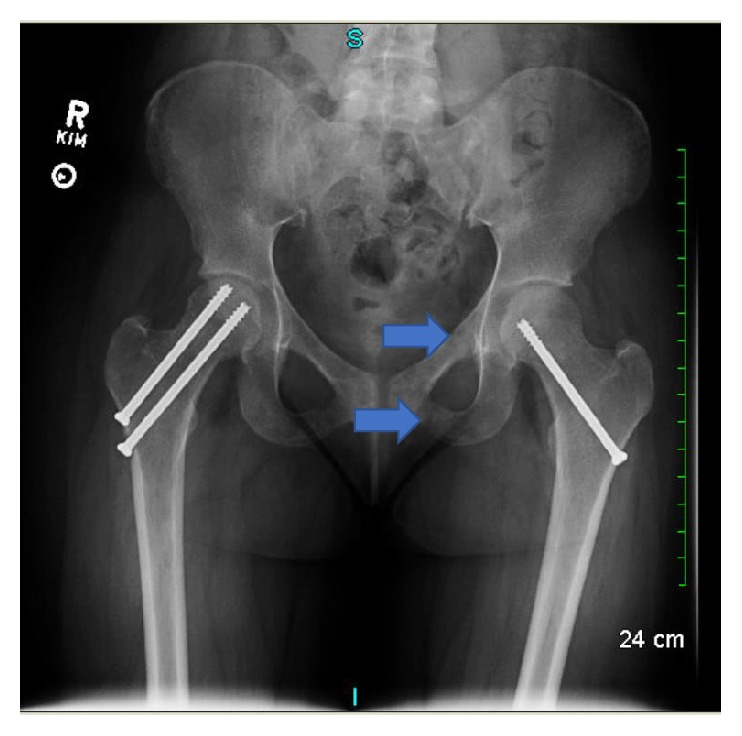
X-ray of the hips and femurs*. New insufficiency fractures (arrows) were noted. There was interval placement of stabilizing screws for the previous right and left femoral neck insufficiency fractures.
